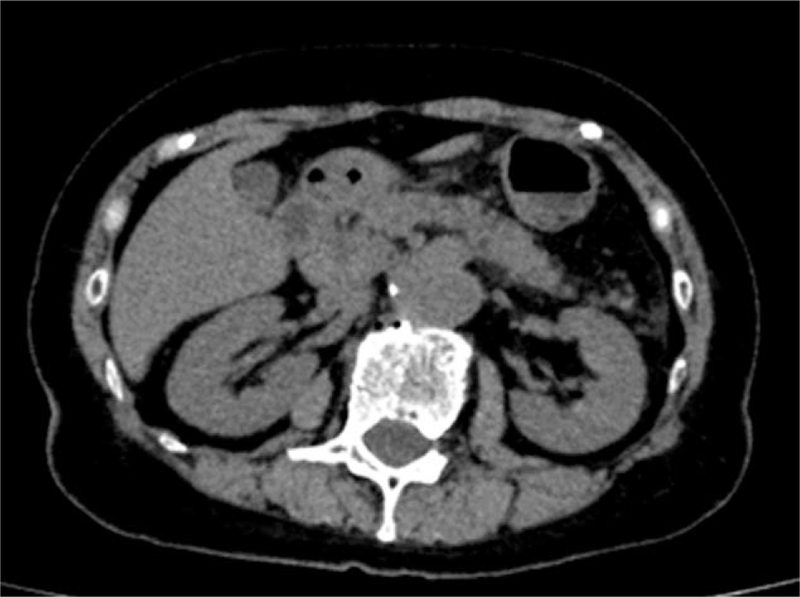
CT scan on day 14 of hospitalization revealed the improvement in the enlargement of the pancreas. CT = Computed tomography.
